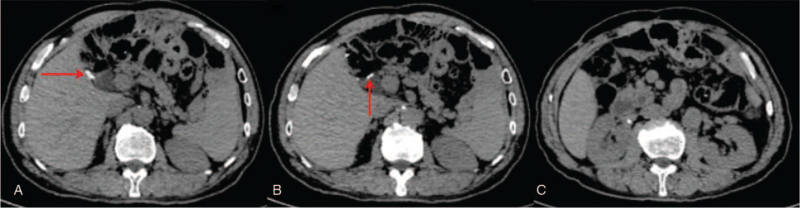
Postoperative computed tomography findings. (A, B) Computed tomography showed that the three clips (red arrow) had not moved. (C) Gradual dilation of the common bile duct (1.2 cm in width) without stone formation at the 12-month follow-up.
